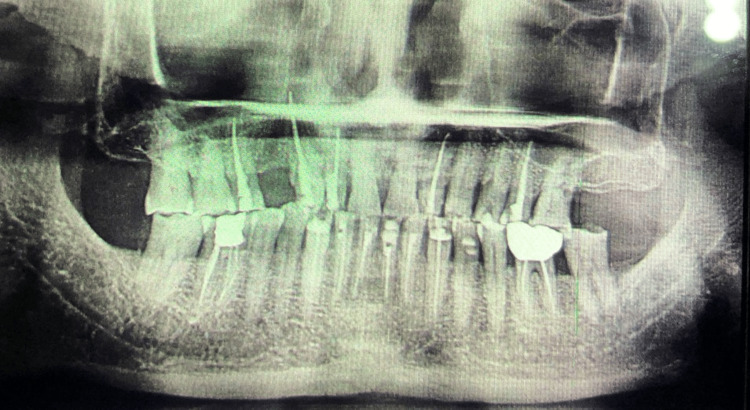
Panoramic radiograph showing the requirement of root canal treatment
